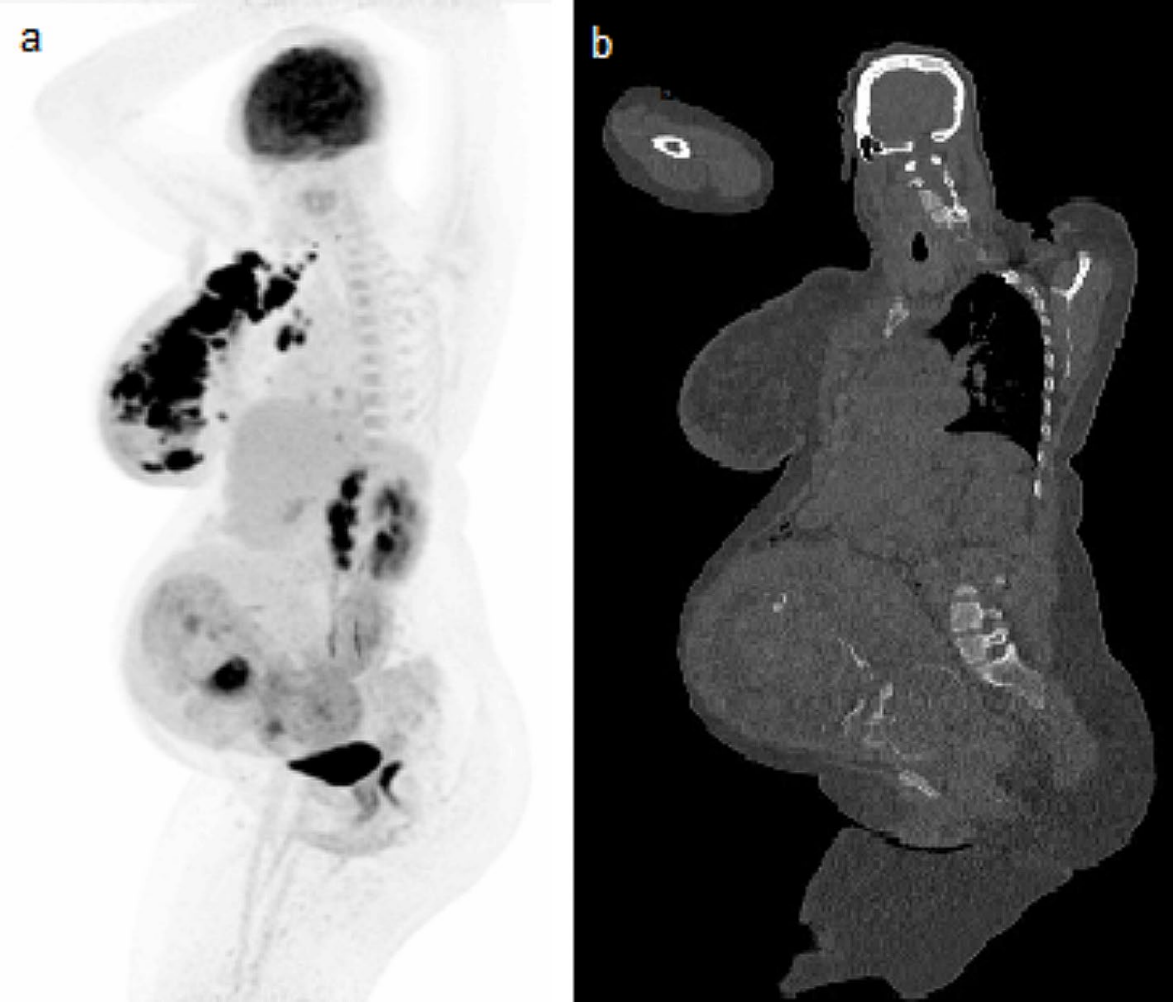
^18^F-FDG PET/CT image including both the mother and the foetus with (a) a Maximum Intensity Projection (MIP) ^18^F-FDG PET image and (b) a slice of the ultra-low-dose CT scan
